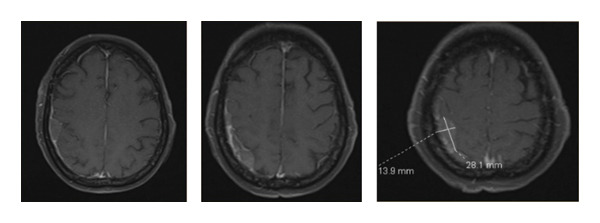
Axial postcontrast T1‐weighted MRI brain obtained one month after intrathecal chemotherapy. MRI demonstrates interval decrease in dural thickening along the frontal and parietal convexities with new nodular dural components along the right parietal and temporal convexities. Background findings include chronic microvascular ischemic disease and multiple cheronic lacunar infarcts.
